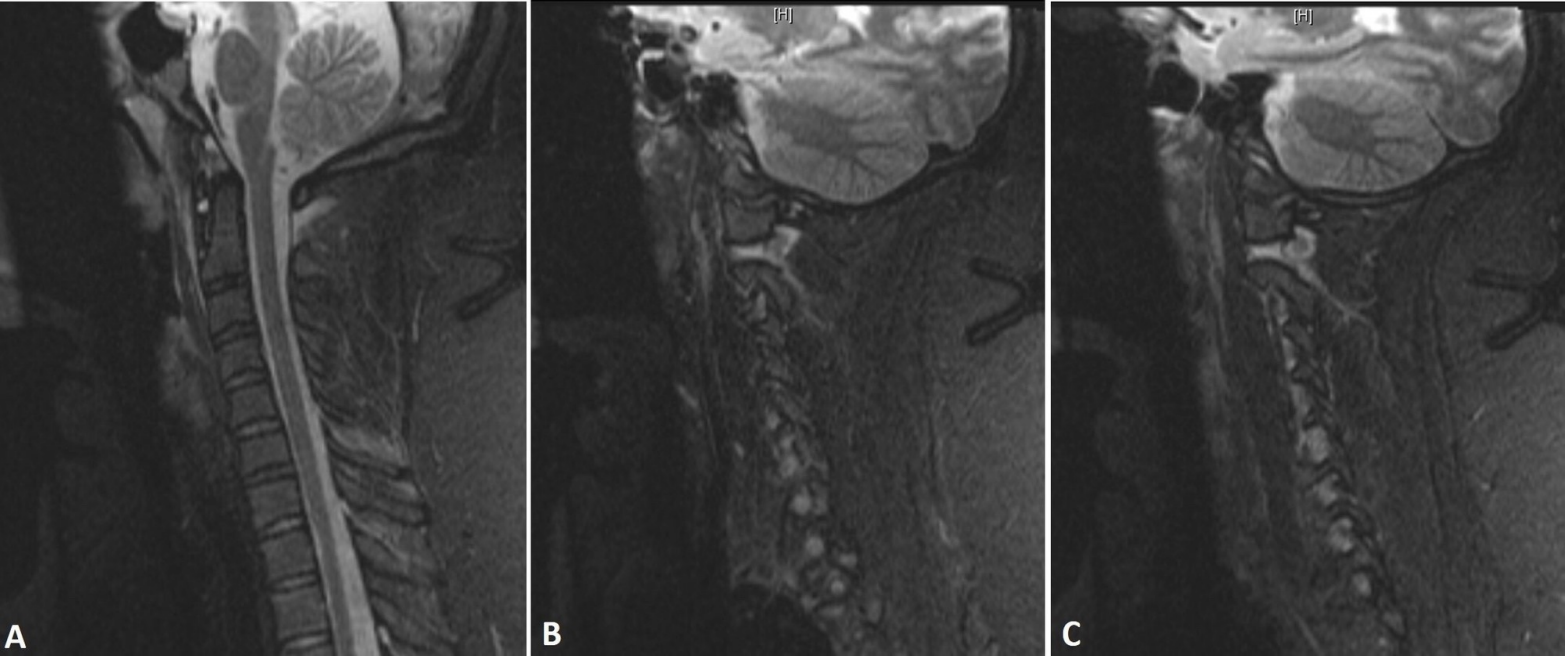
Ligamentous disruption Sagittal (A) and bilateral parasagittal (B and C) STIR MRI sequences showing disruption of the apical ligament and abnormal BDI (A) as well as increased signal intensity at the level of the atlanto-axial joints with distraction and an increase in signal intensity at the level of the condyle C1 joints bilaterally (B and C). STIR: short tau inversion recovery; MRI: magnetic resonance imaging; BDI: basion-dens interval.
